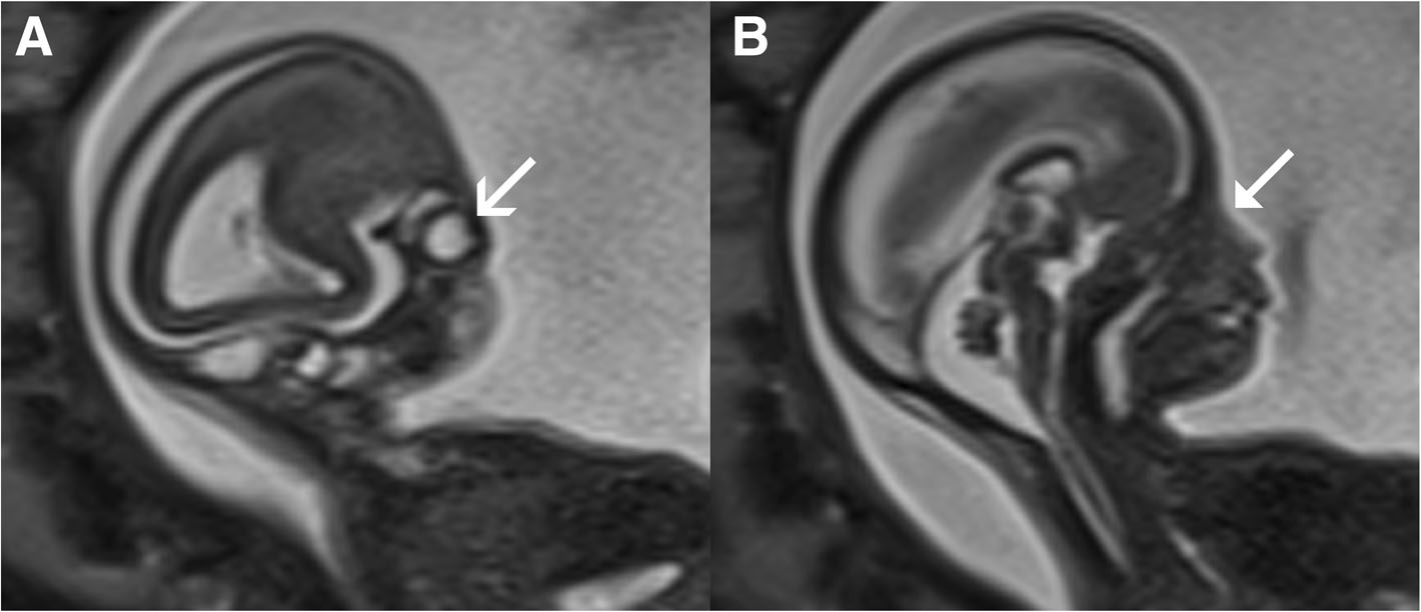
Magnetic resonance images of the fetus with anophthalmia, showing parasagittal views of the fetal head. a The normal eye; b anophthalmia. The *triangular arrow-head* represents anophthalmia. The *simple wide arrow-head* represents the normally formed eye
